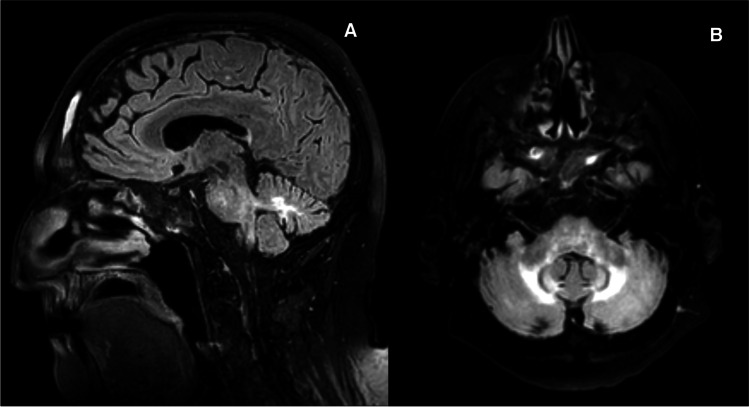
Magnetic resonance imaging (FLAIR sequence) showing increased signal in the deep white matter (A sagittal, B axial) of both cerebellar hemispheres with some less prominent involvement of the cerebellar peduncles and brainstem
